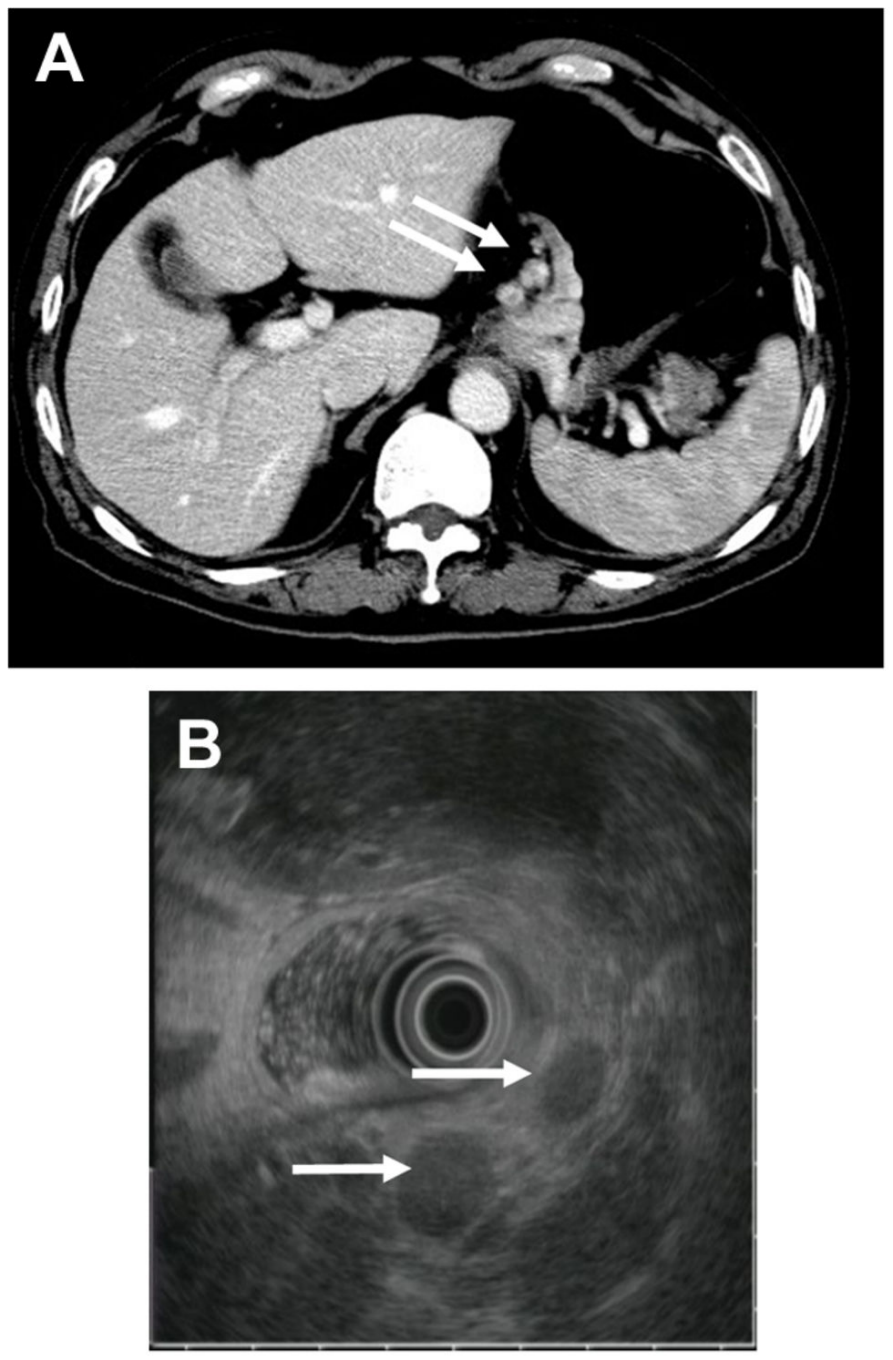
N staging using MSCT and EUS. A. MSCT- Lymph node metastases: Transverse CT image of two enlarged lymph nodes (arrows) near the left gastric artery, which shows strong enhancement on enhanced scanning and areas of necrosis in the nodes. B. EUS- Lymph node metastases: EUS showing two hypoechoic lymph nodes (arrows), the largest of which is 16.2×14.3 mm.
